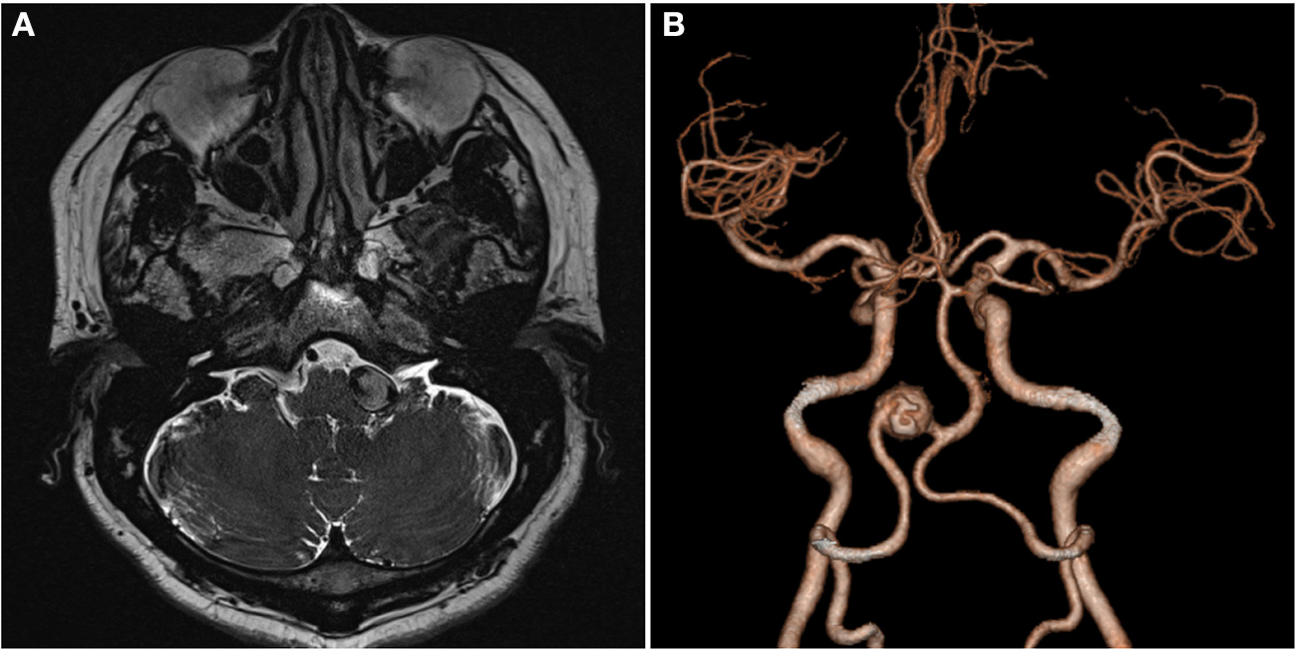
Images of the patient upon admission. (A) Axial T2-weighted MR image shows a fusiform aneurysm as a flow void signal left of the pons. (B) Three-dimensional CT angiography shows VA fusiform aneurysm. VA, vertebral artery.
